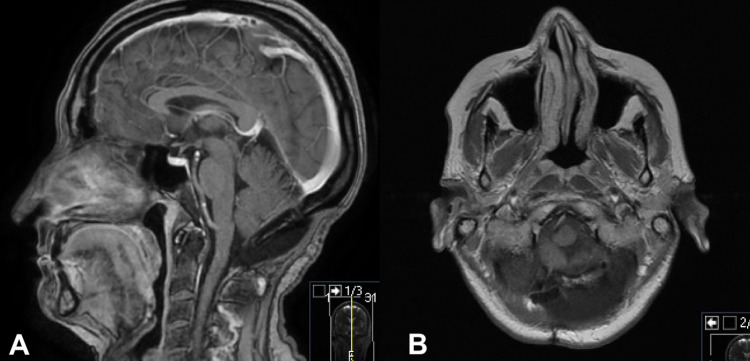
Postoperative MRI scan. The scans in sagittal (A) and axial (B) planes showing gross-total resection.
